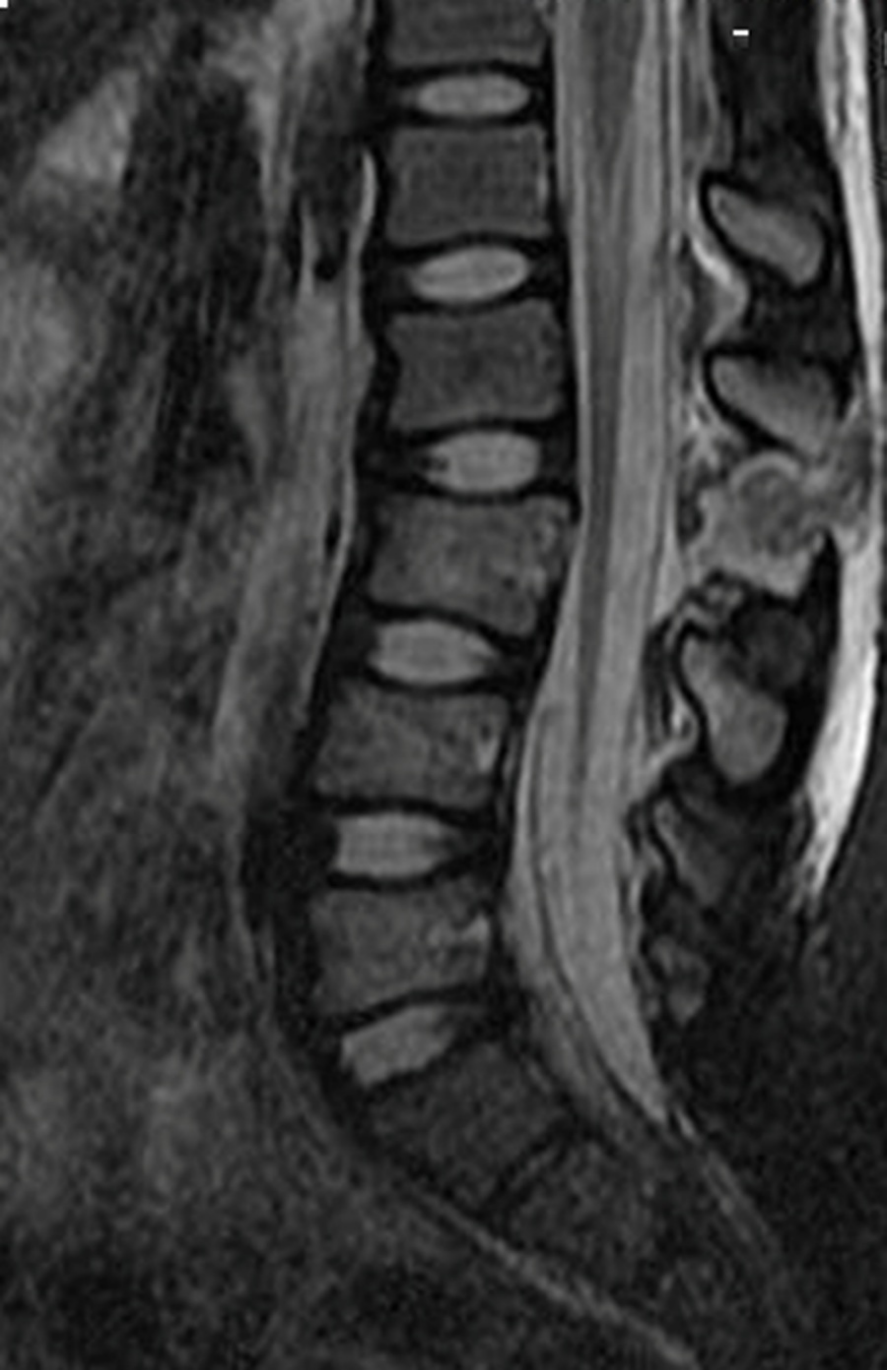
T2 weighted MRI of the lumbar spine without contrast revealing a hyperflexion injury with rupture of the interspinous ligaments and ligamentum flavum at the L2-L3. The increased signal intensity is consistent with the formation of a hematoma sac.
